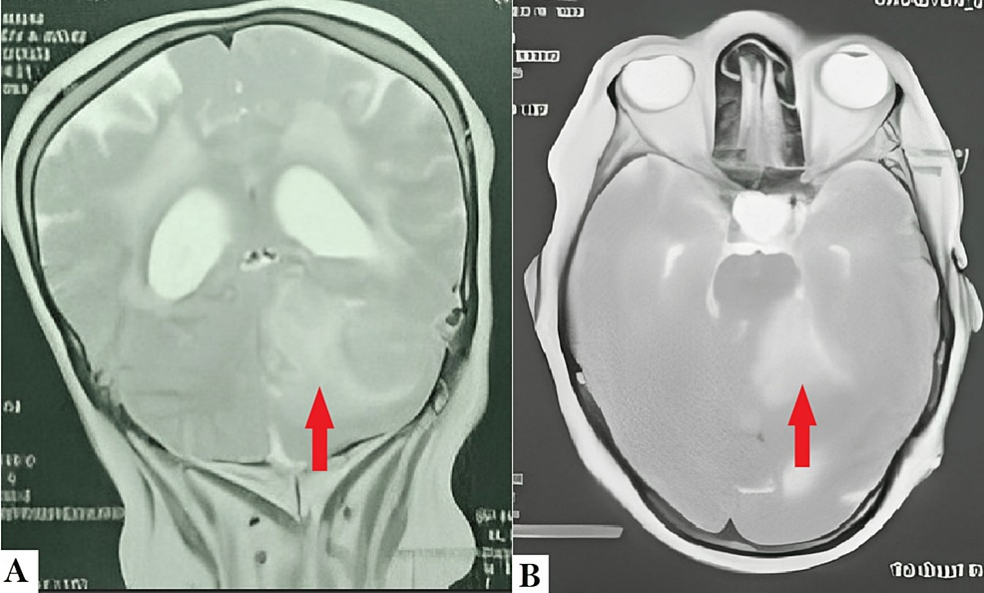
Cerebral MRI of the patient showing a left cerebellar pontine angle lesion responsible for obstructive tri-ventricular hydrocephalus. (A) Cerebral MRI in T2 sequence, coronal plane, showing a left cerebellar lesion with peripheral edema (red arrow) exerting a mass effect on the surrounding structures as well as obstructive hydrocephalus due to the compression of the fourth ventricle. (B) Cerebral MRI in flair sequence, axial plane, showing the same lesion centered on the left cerebellar pontine angle (red arrow) with important peripheral edema exerting a mass effect on the surrounding structures.
